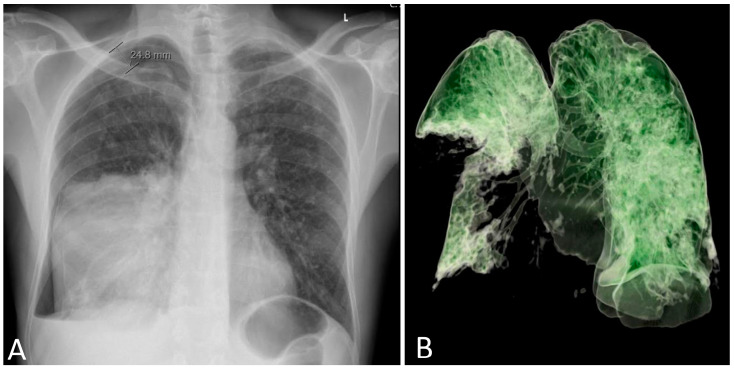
Chest radiography on hospital admission with right-sided pneumothorax and a pulmonary infiltrate of the medial and lower lobe of the right lung (A). A complete absence of aeration of the lower and medial right lobe was present on day 3 (B). L: left side.
